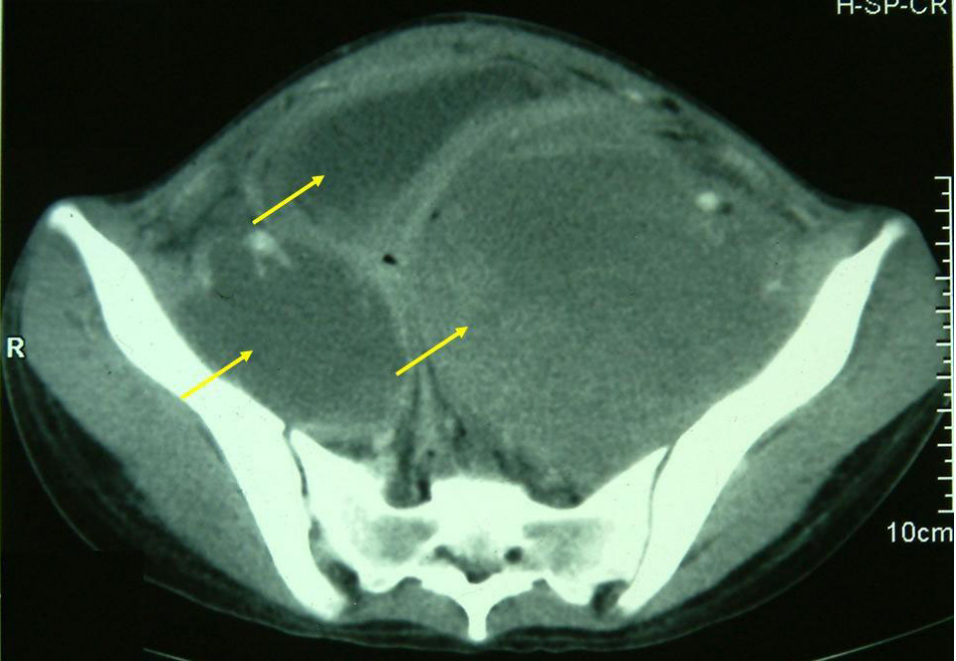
CT scan showing the tumor extensions.
